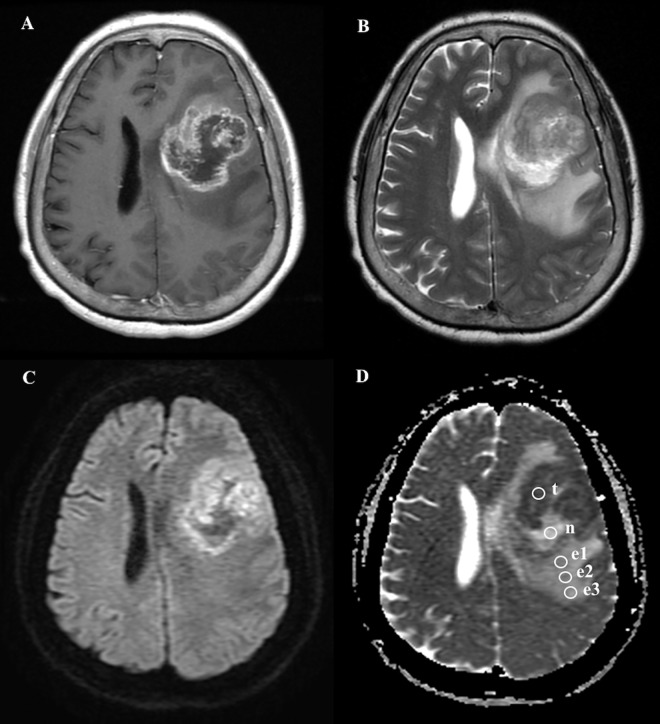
A 64-year-old female with pathologically-proven glioblastoma multiforme after biopsy. (A) Axial contrast-enhanced T1WI showed a tumor mass with rim enhancement and central hypointense non-enhancing necrosis in the left frontal lobe. (B) Axial T2WI showed extensive peritumoral edema associating with the mass lesion. (C) DWI showed a ring-shaped hyperintense tumor mass, central hypointense tumor necrosis, and isointense peritumoral edema. (D) ADC map showed ROIs (circles) in the tumor necrosis (n), the most strongly-enhanced tumor area (t), and the gradient of peritumoral edema from the most proximally-located area (e1) of the peritumoral edema to the most peripheral location (e3). Measured ADC (b = 1000 sec/mm^2^) in the tumor necrosis (ADCn) is 1.85 x 10^−3^ mm^2^/s; the most strongly-enhanced tumor area (ADCt), 0.87 x 10^−3^ mm^2^/s; proximal peritumoral edema (ADCe1), 1.3 x 10^−3^ mm^2^/s; middle peritumoral edema (ADCe2), 1.36 x 10^−3^ mm^2^/s; distal peritumoral edema (ADCe3), 1.43 x 10^−3^ mm^2^/s.
